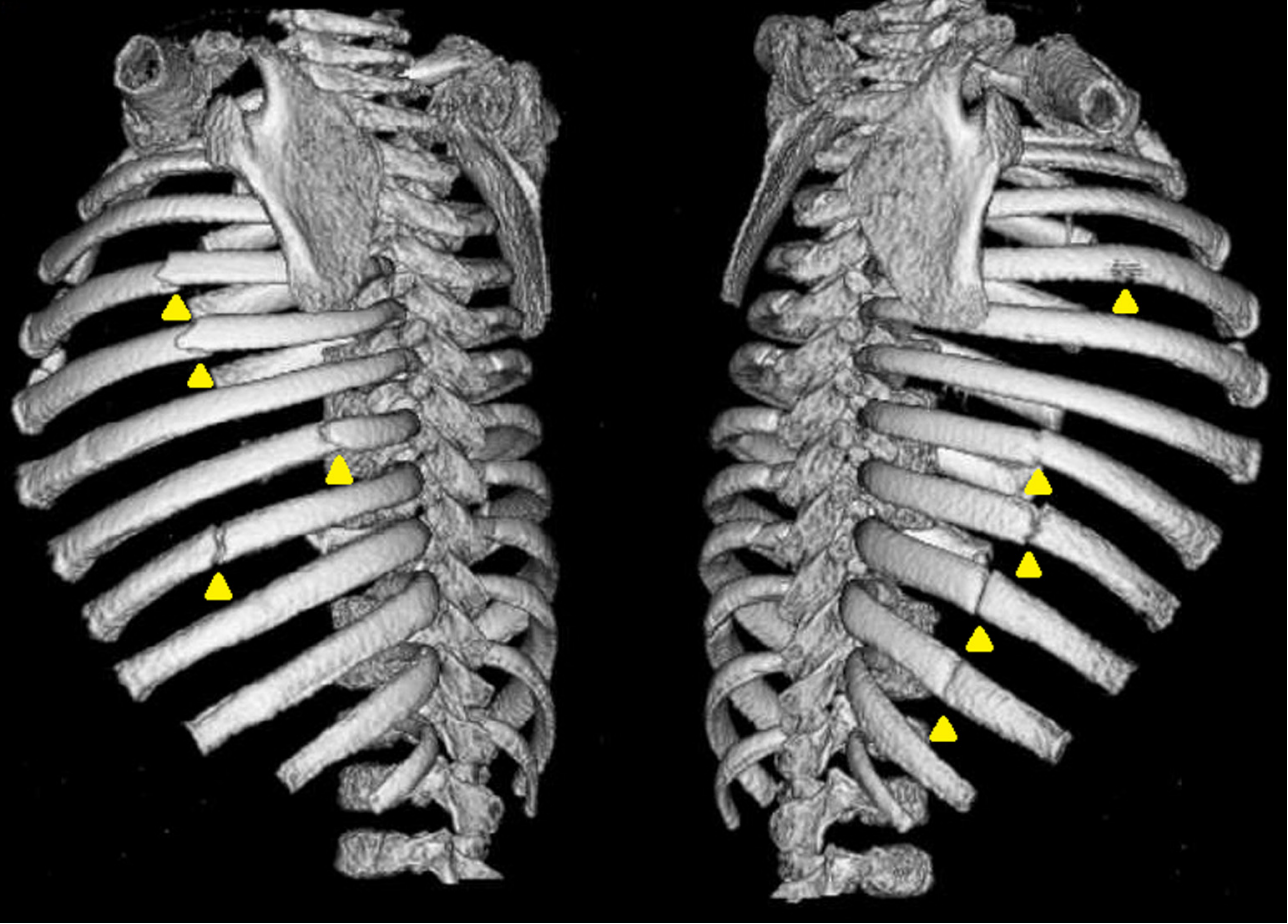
3D chest computed tomography of bone shows multiple posterolateral and posteromedial rib fractures.
